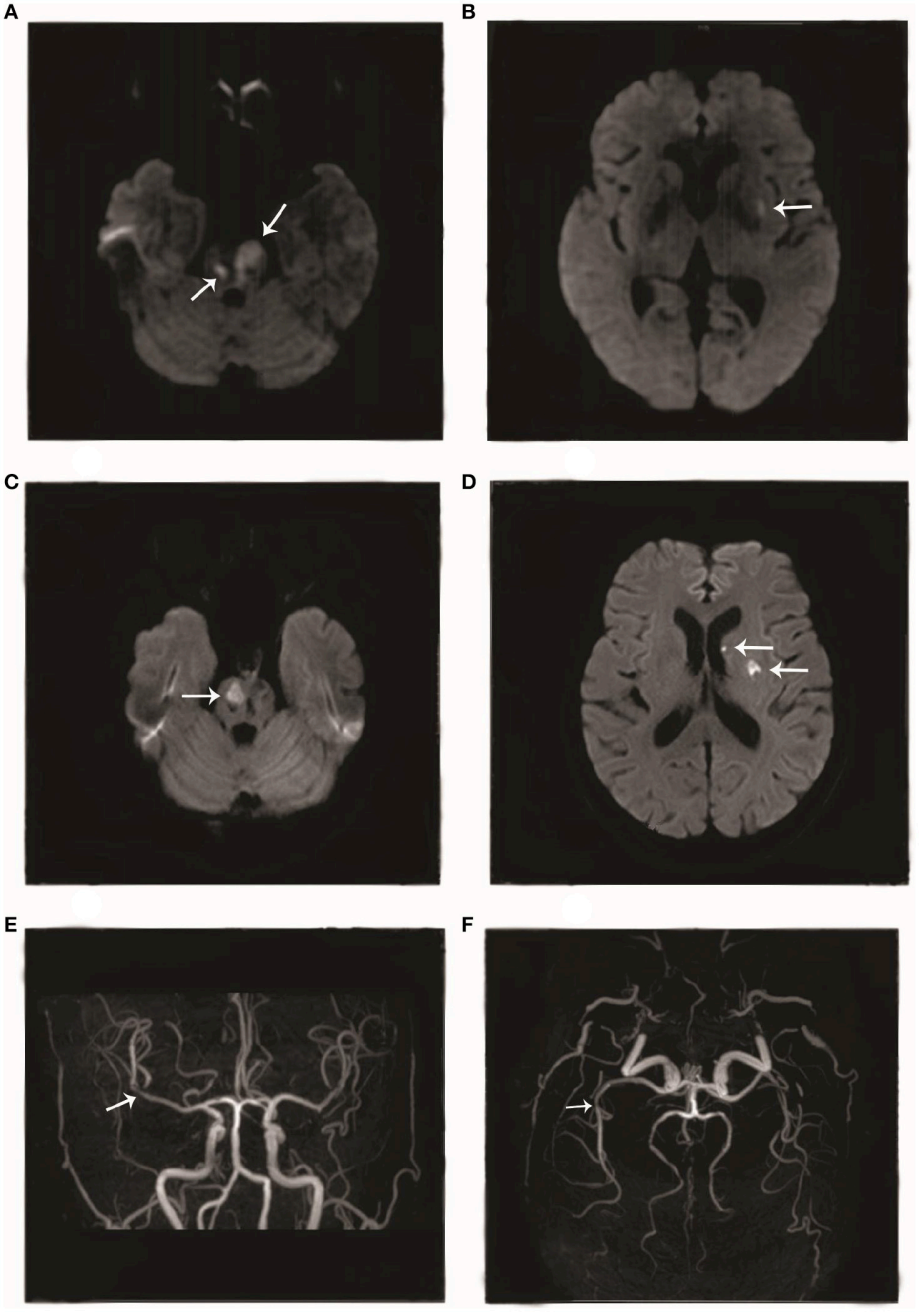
Imageological changes before admission. Diffusion-weighted imaging (DWI) performed in September 2017 showed hyperintense areas in the (A) right and left pons and (B) left insular cortex. DWI performed in November 2017 showed hyperintense areas in the (C) right pons and (D) left putamen. Magnetic resonance angiography performed in November 2017 showed (E, F) mild stenosis in the right middle cerebral artery.
